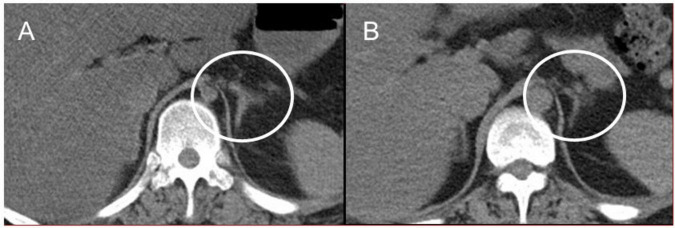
Abdominal CT images. (A) Postmortem CT imaging reveals hyperdensity in the left adrenal gland (indicated by a circle), suggestive of adrenal hemorrhage. (B) A CT scan performed 5 months prior to admission shows no abnormalities in the left adrenal gland (indicated by a circle). CT, computed tomography.
